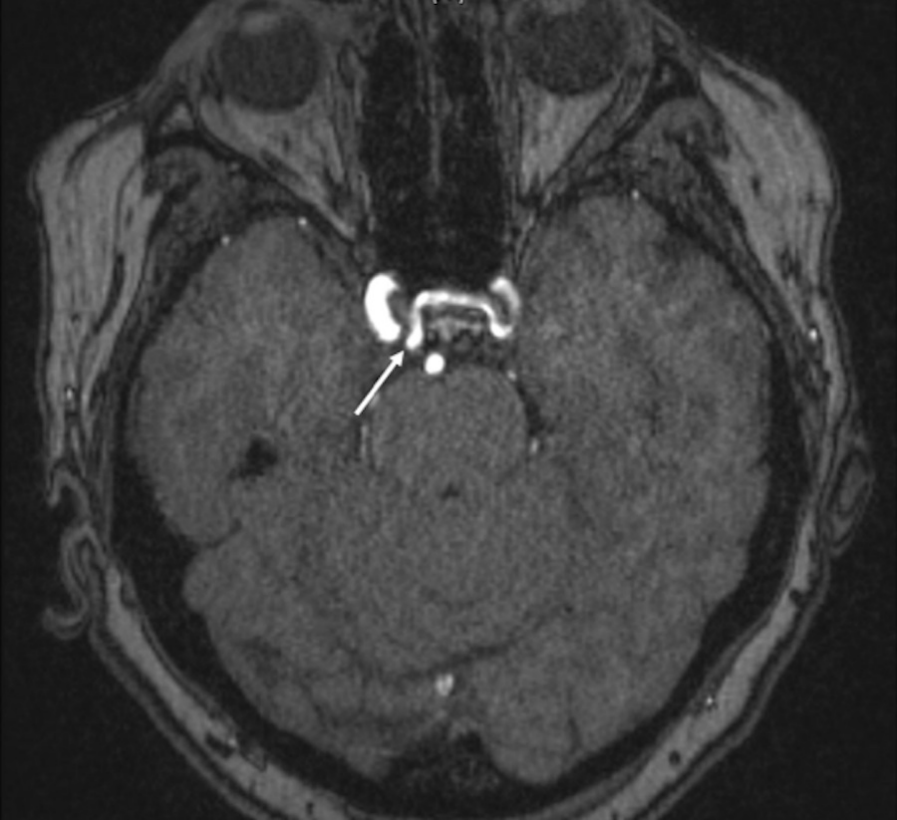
Axial 3D time of flight MRI of the head, noting the unusual origin of the left internal carotid artery (arrow) from the contralateral cavernous segment of the right internal carotid artery. Note the course of the left internal carotid artery through the floor of the sella turcica.
